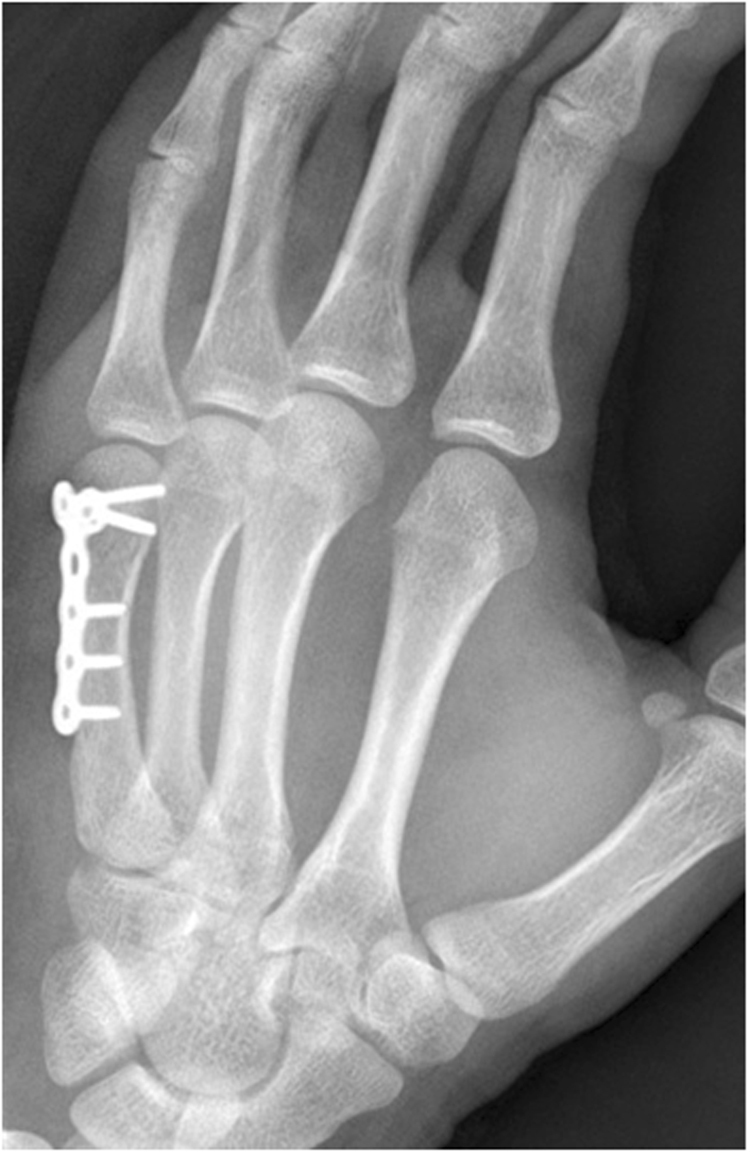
A representative radiograph after locking plate fixation.
